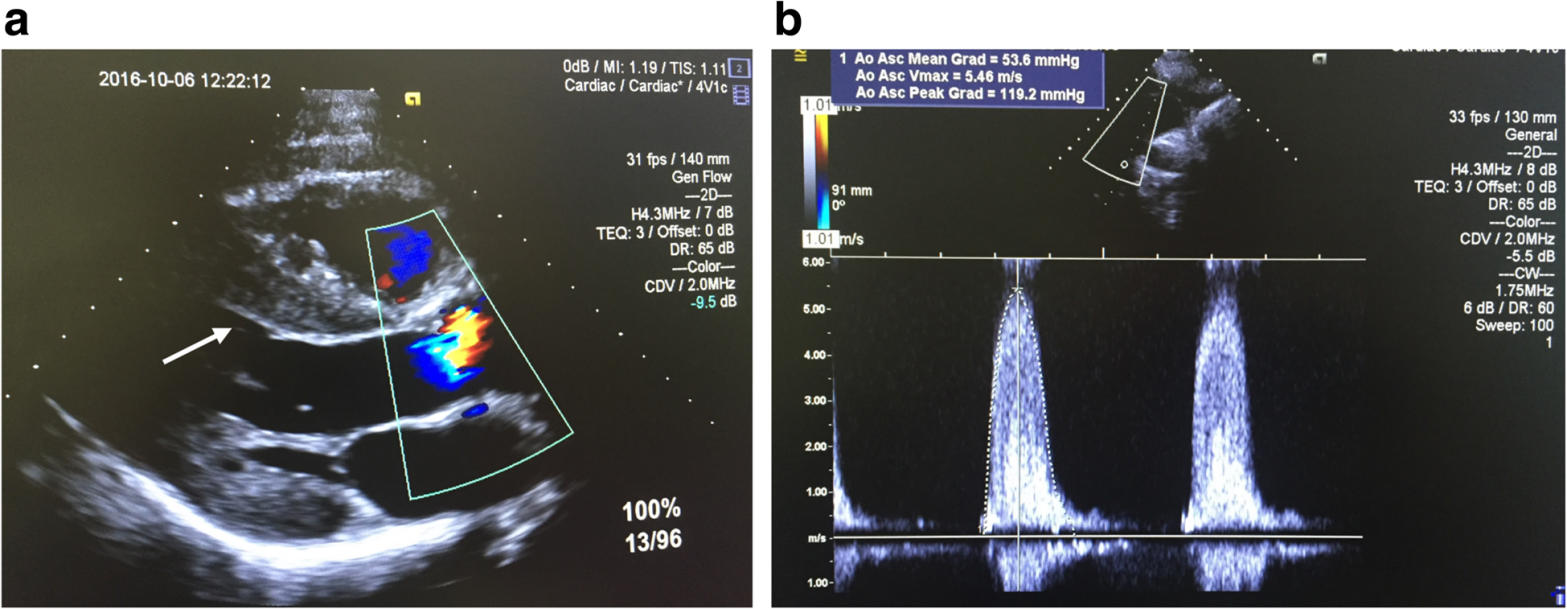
a Echocardiography demonstrating an increased septal diameter (arrow). b Echocardiography measurements demonstrating a mean gradient of 54 mmHg, maximal gradient of 119 mmHg, and peak flow of 5.5 m/s through the aortic valve orifice
